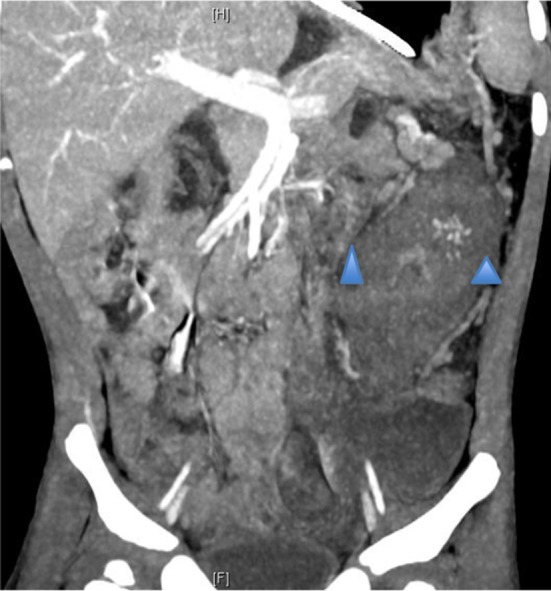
Abdominal CT with contrast. Marked bowel wall thickening of left colon (see blue arrows) extending from the distal transverse to the descending colon. There is an area of spared bowel in the mid descending colon. The thickened bowel is homogenous in attenuation, with relative hyper enhancement of the mucosa. There is abrupt transition between normal and abnormal bowel (shouldering). There is involvement of the serosa with multiple serosal nodules and infiltration of the pericolonic fat.
